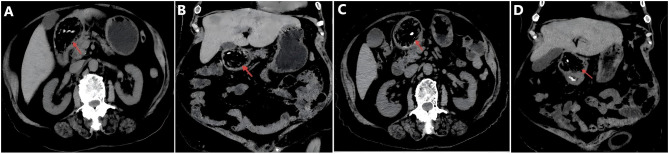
Abdominal computed tomography images. (A, B) Axial and coronal CT images showing a large-sized bezoar in the duodenal bulb. (C, D) Axial and coronal CT images after 3 days of chemical dissolution treatment.
